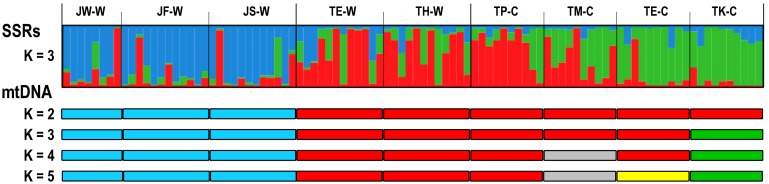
Structure analysis of small abalone populations. Estimated population structure based on the highest probability STRUCTURE run at K = 3 by using SSRs data. Each individual is represented by a thin vertical line, which is partitioned into K colored segments that represent the individual’s estimated membership fractions in each of the K clusters. Cluster 1 = blue, cluster 2 = red, cluster 3 = green. Estimated population structure based on mtDNA COI sequences by using the highest probability SAMOVA (*F*_*CT*_) run from K = 2 to K = 5 (S6 Table). JW-W, JF-W, and JS-W: wild population from Wakayama, Fukuoka, and Shimonoseki, Japan, respectively. TP-C, TM-C, TE-C, and TK-C: cultured stocks from Penghu Island, Miaoli, Keelung, and Kaohsiung, Taiwan, respectively. TH-W and TE-W: wild population from Keelung and Hualien, Taiwan, respectively.
